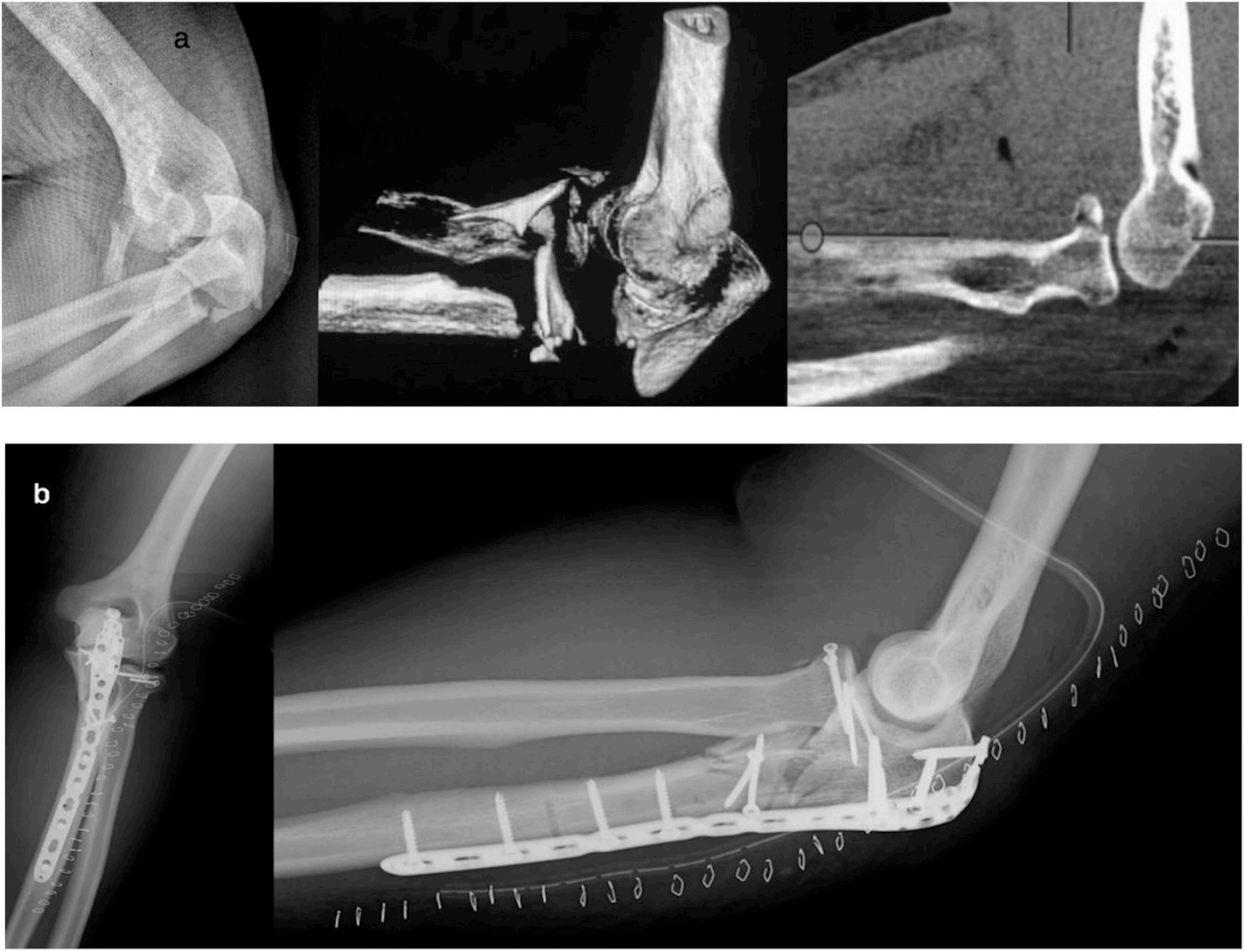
a) Radiographs and CT scan: comminuted fracture of the proximal ulna and coronoid process. Simple fracture of the radial head in a 26-year-old man. b) Radiographs at 9 months after surgery: global posterior approach and subcutaneous Kocher approach – Pre-contoured locking compression plate, two lag 2.7 mm screws and two loose K. wires for synthesis of comminuted coronoid process. Synthesis of the radial head with 2 small fragments screws (2.4 mm). Fracture healing; presence of heterotopic ossifications.
